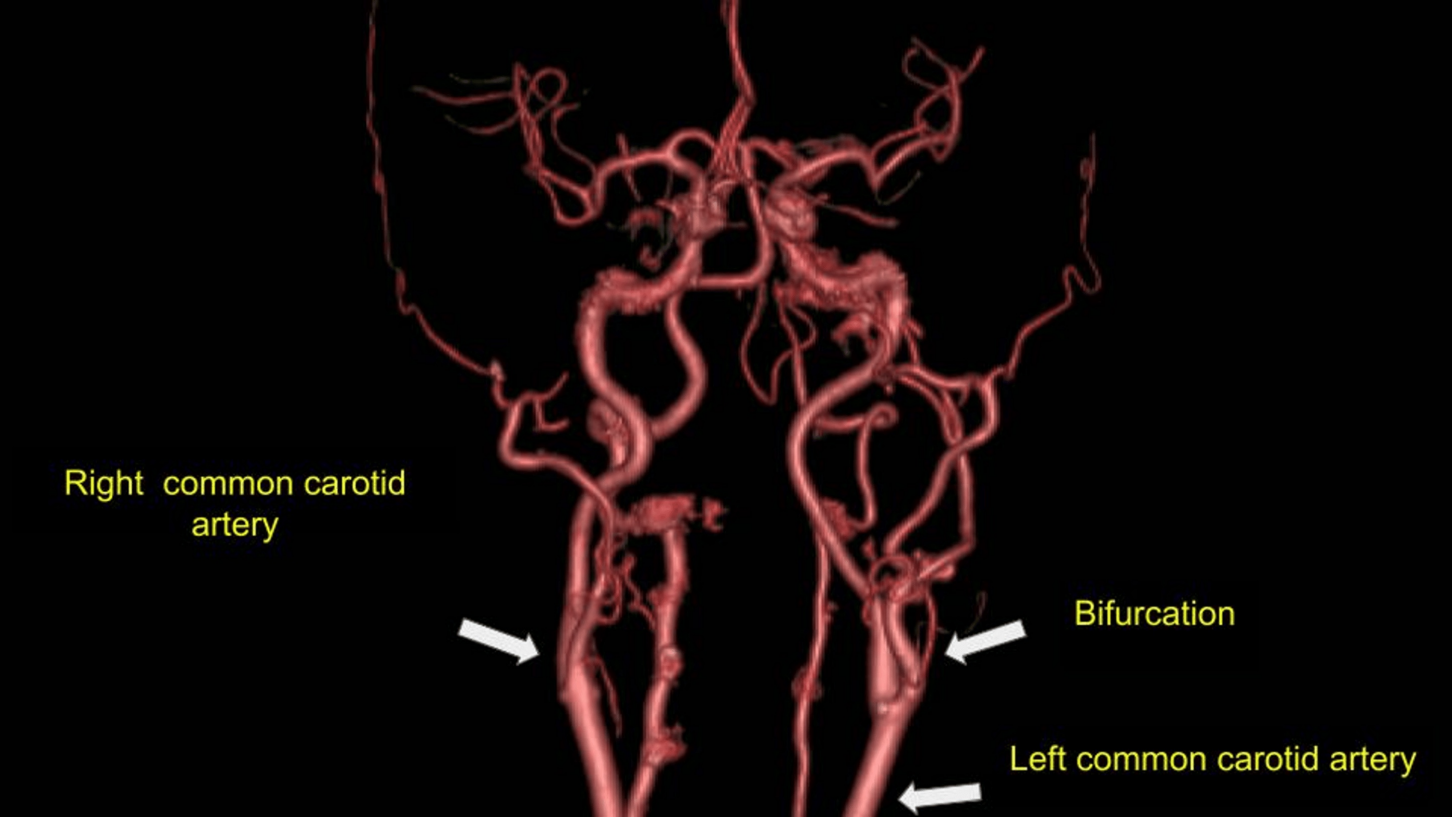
CT: 512 slices were used in single phase, and after administration of non-ionic intravenous contrast medium (vol. 50 mL), there is no evidence of solid hypervascular lesions in the right carotid bifurcation Image credit: Authors
